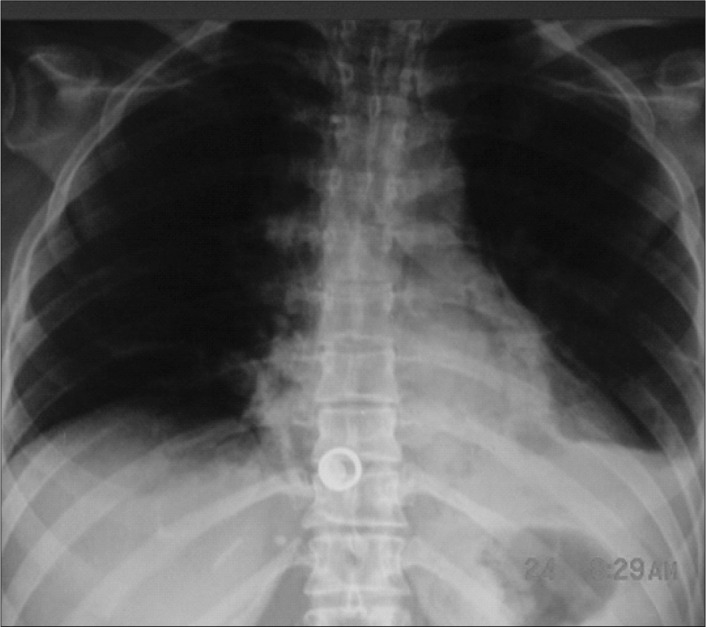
Chest x-ray showing left pleural effusion and pneumomediastinum in a woman operated for morbid obesity.
